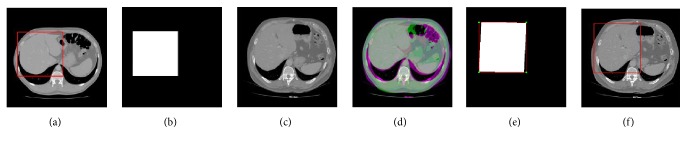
An example of liver positioning using ROP method. (a) A CT slice with the closest average liver volume and liver's bounding box region (red). (b) The binary image. (c) Another image from different patient. (d) The corresponding image registration result. (e) Four new vertexes (green) based on the transformation result. (f) Obtained liver positioning result.
